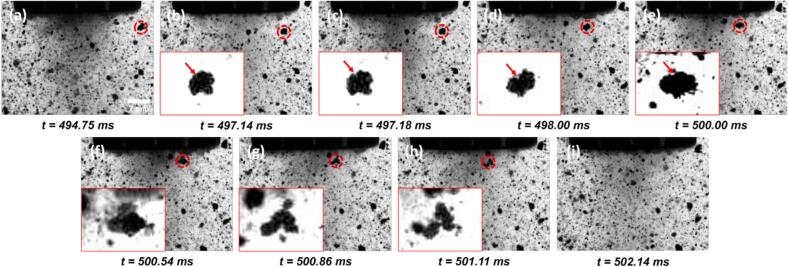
Agglomerate fragmented in the CZ of TX-100 solution during UST. The inset of the figure with the adjusted brightness and contrast shows the zoomed in image of the MWCNT agglomerate labelled by red dotted circle. The red arrows in the figures indicate the positions of defects where the bubble nucleated on the agglomerate. Time values represent the elapsed time after the onset of ultrasonication. (Supplementary Video 1 (SV1)). (For interpretation of the references to colour in this figure legend, the reader is referred to the web version of this article.)
